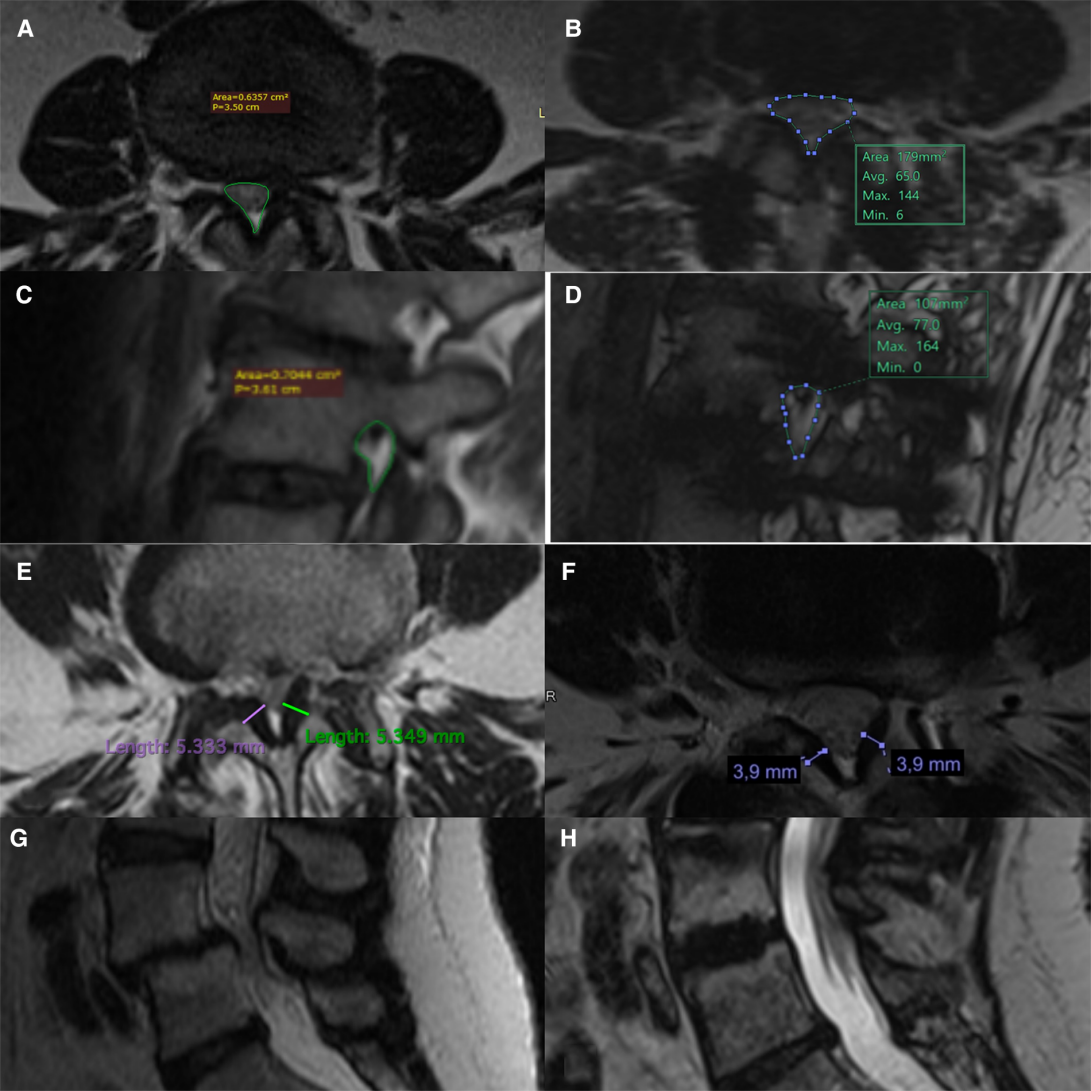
Pre- and post-operative comparison on MRI scans. Measurement of pre and post-op central canal area (A,B), foramen area (C,D), thickness of the yellow ligament (E,F). Example of reduction of degenerative spondylolisthesis through indirect LLIF decompression technique (G,H).
